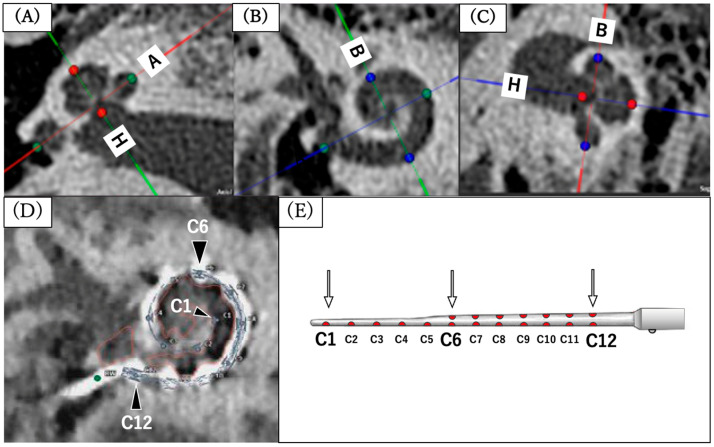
Measurement of cochlear parameters and electrode localization using CT-based analysis (OTOPLAN). (A–C) Measurement of cochlear basal turn diameter (A), width (B), and cochlear height (H). (D) Electrode localization and angular insertion depth. (E) FLEX28 electrode array and electrode numbering.
